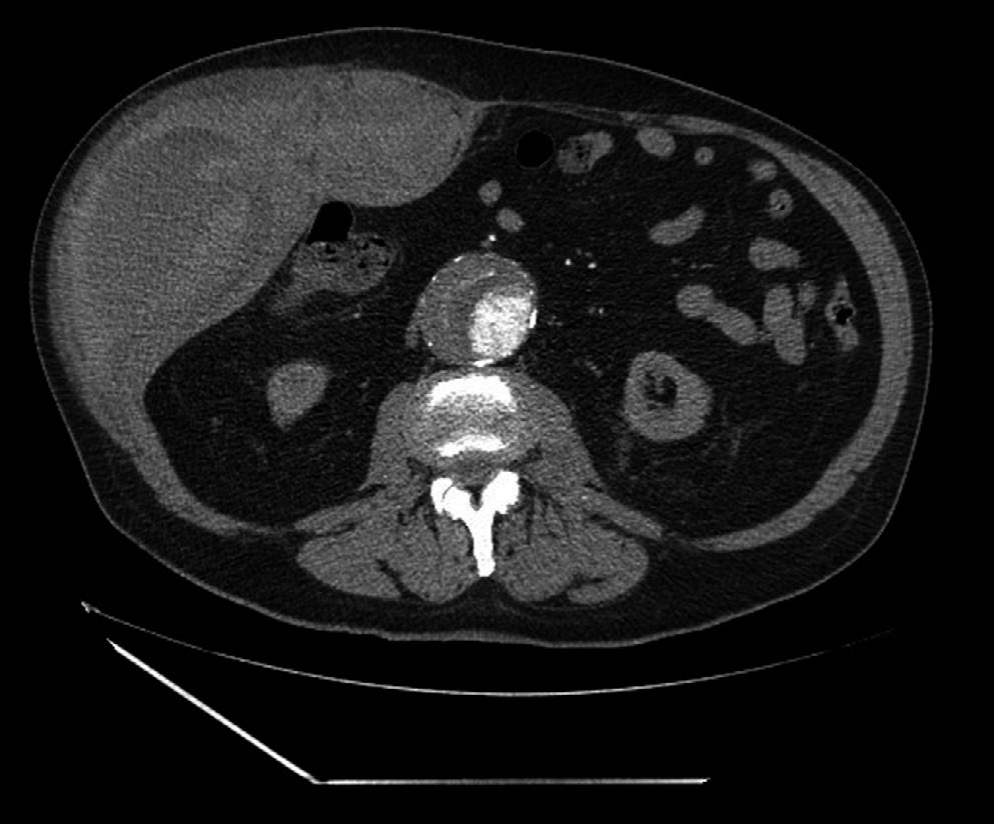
Case 4: CT scan of the abdomen and pelvis revealing a right rectus sheath hematoma. Massive nodular formation (heterogeneous), with solid and liquid areas, compatible with large hematoma depending on the anterior abdominal wall and right side
